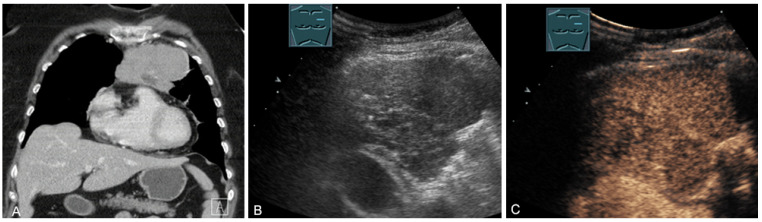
A patient with tumor formation in the anterior mediastinum on CT (provided by Prof. Dr. Andreas H. Mahnken, Marburg, Germany) (A), B-mode US (B), and CEUS (C), surgically confirmed as malignant thymoma stage B1.
